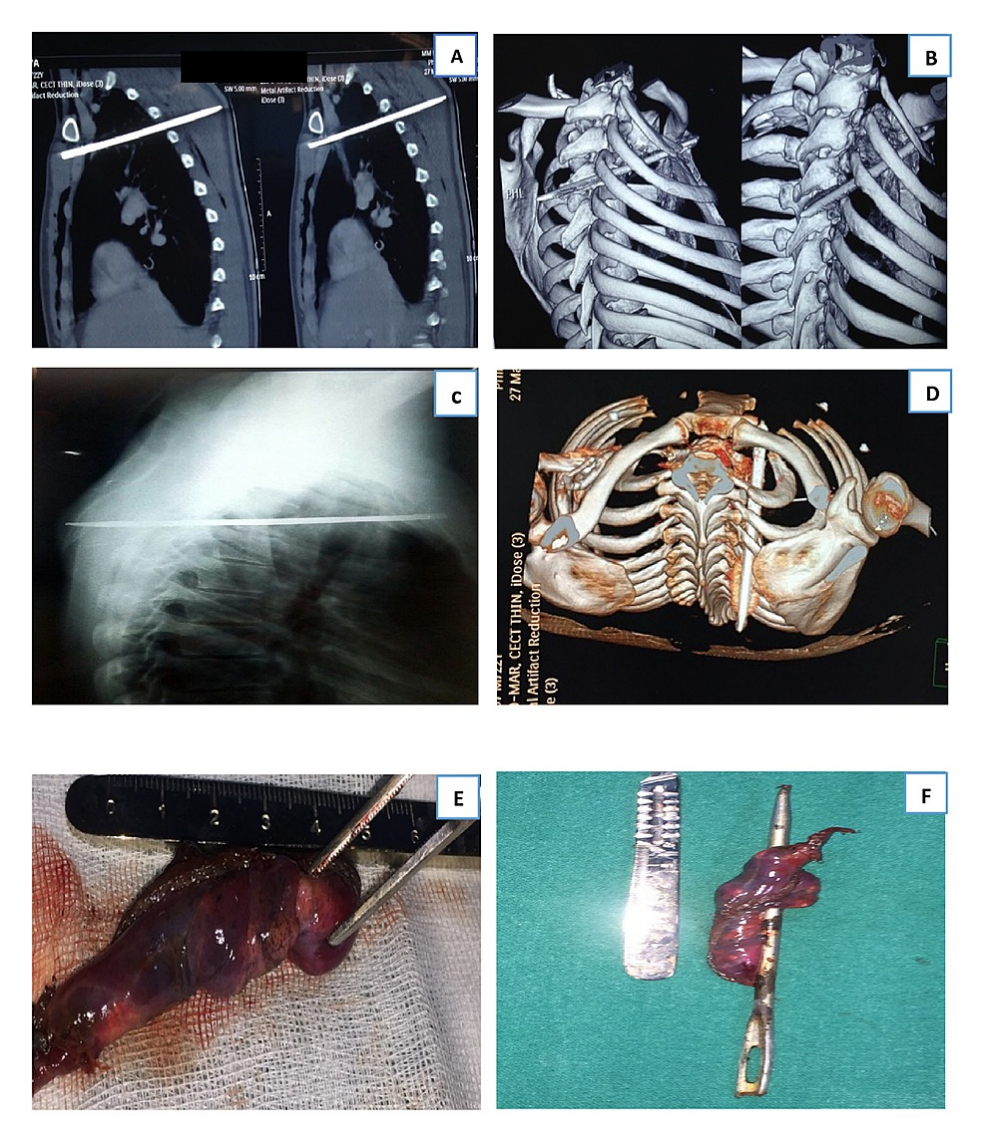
CT scan of the chest (A, B, D) showing spindle piercing the right anterior chest wall into the apical segment of the right lung and through the posterior chest wall. X-ray chest showing the same spindle passing through and through the right lung apex. The spindle after being removed from the body by surgical exploration (E, F).
